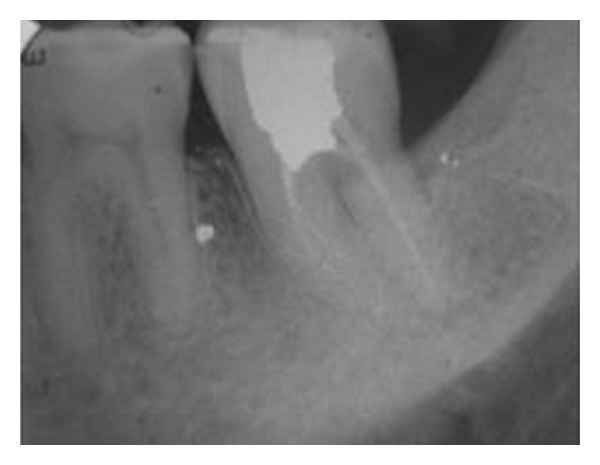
1-year recall radiograph.
